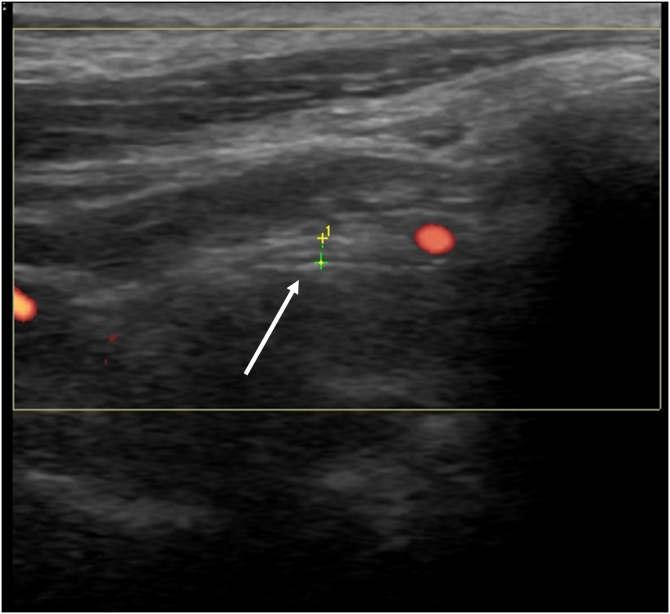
Ultrasound imaging/measurement (longitudinal view) of the facial nerve (white arrow) as they exit the stylomastoid foramen.
